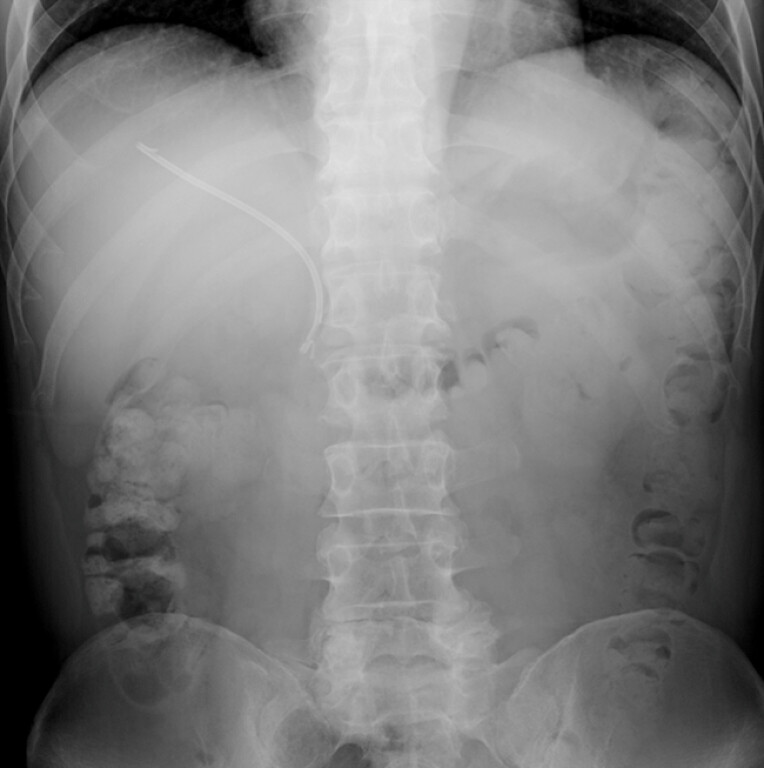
Abdominal radiograph showing the migrated plastic stent.
